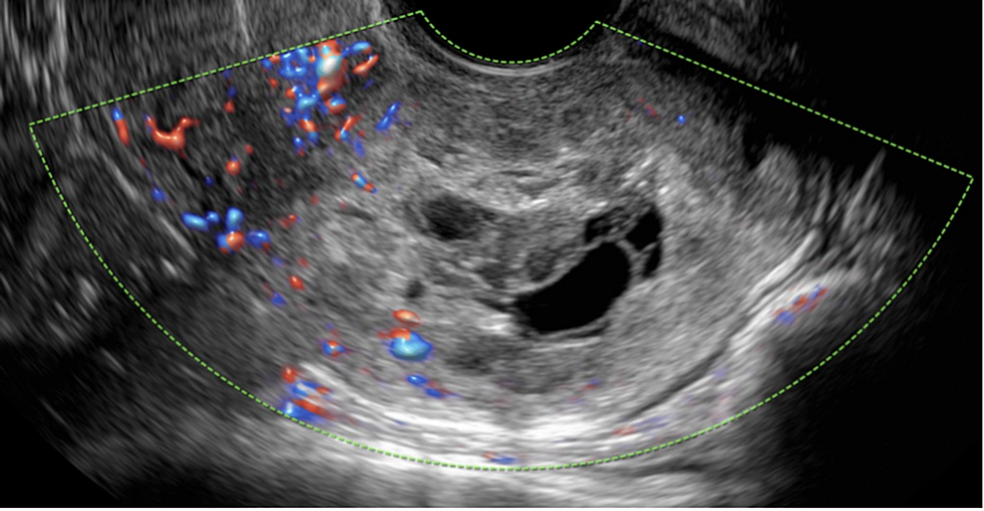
Transvaginal pelvic ultrasound following multi-dose methotrexate regimen and uterine artery embolization.
